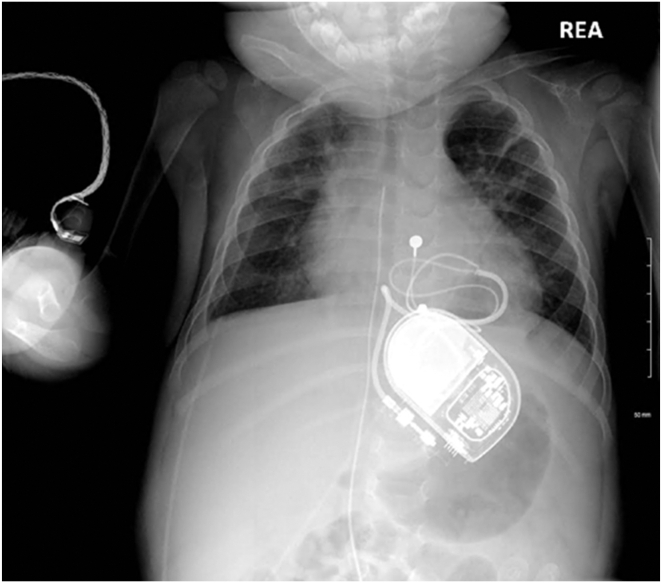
Chest radiograph showing the single-chamber ventricular epicardial lead and pacemaker device.
